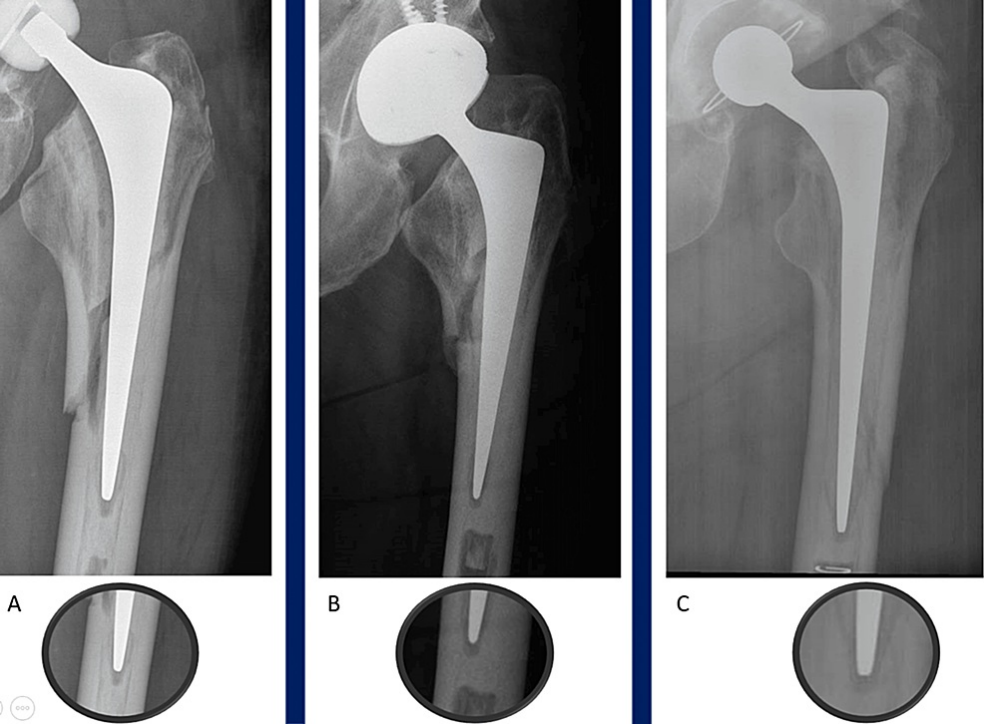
SINC negative periprosthetic femoral fractures AP radiographs of three separate patients (A)-(C) demonstrating periprosthetic hip fractures through the cement mantle with a negative SINC sign. SINC: subsidence-in-centraliser.
